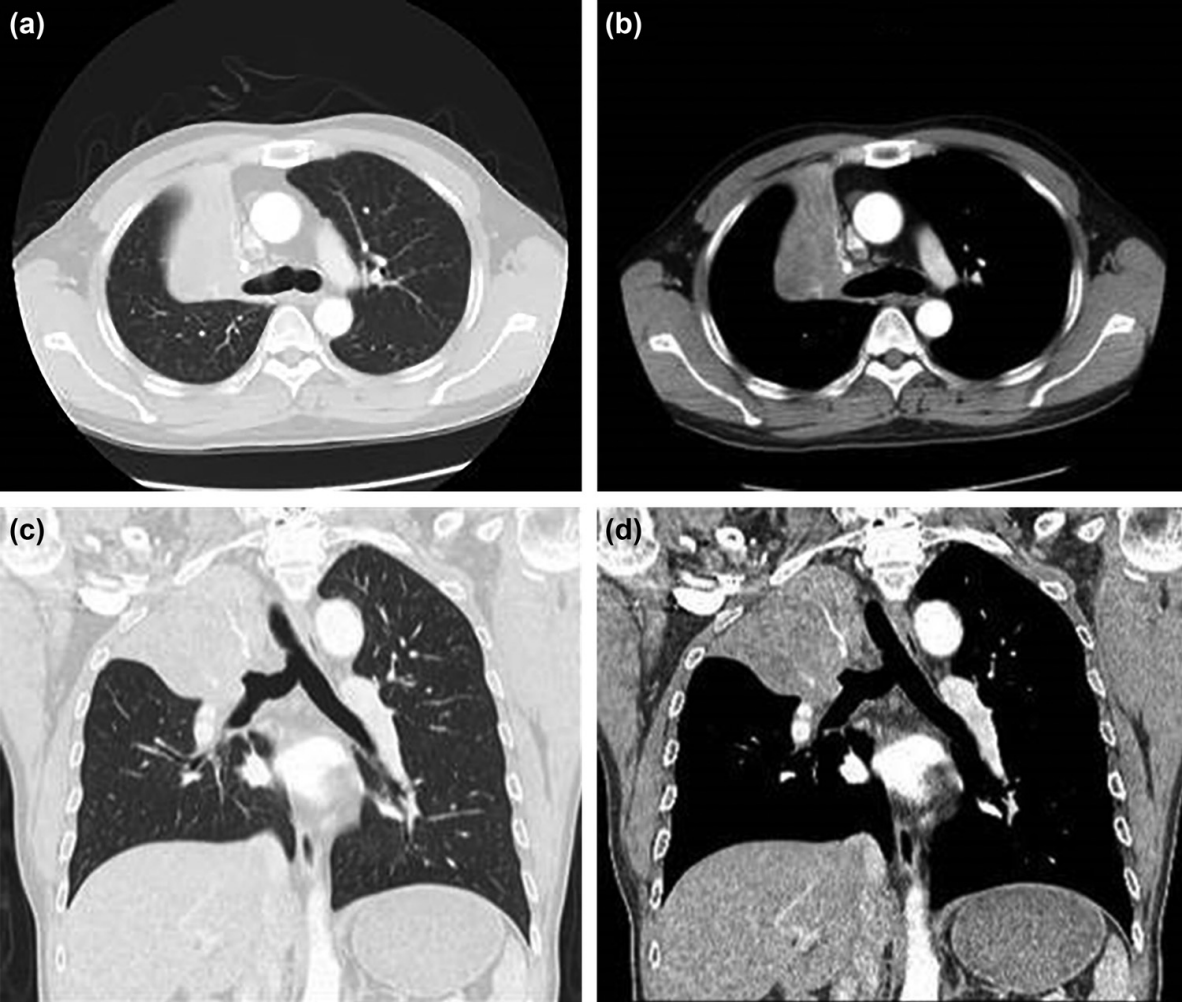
Chest CT scan of the right upper lobe of the bronchus. The right upper lobe bronchus was occluded and the surrounding soft tissue mass was about 7.9 cm × 6.7 cm × 6.0 cm.
